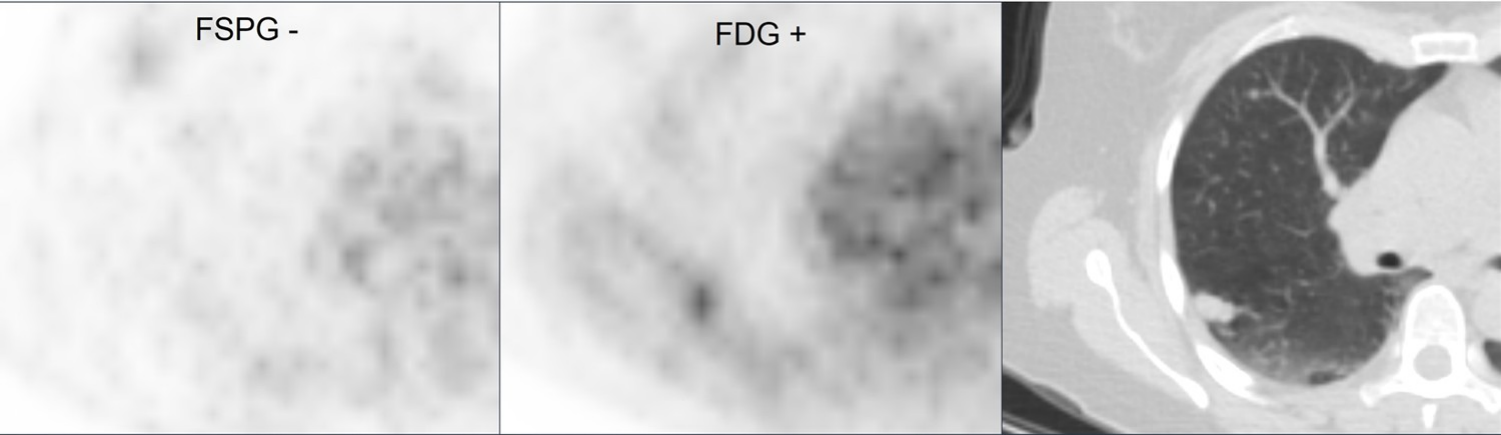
Subject with negative ^18^F-FSPG (SUVmax 0.9) and positive ^18^F-FDG (SUVmax 2.4) PET/CT.
